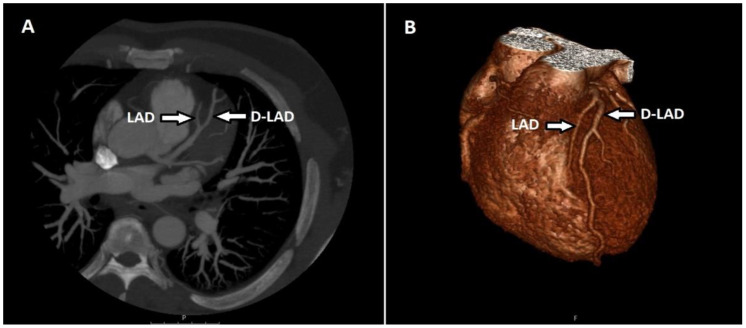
Maximum-intensity projection (MIP) of axial CT (A) and volume-rendered (VR) (B) image shows the LAD terminating above the atrioventricular groove and the duplicated LAD (D-LAD) extending distally along the groove.
